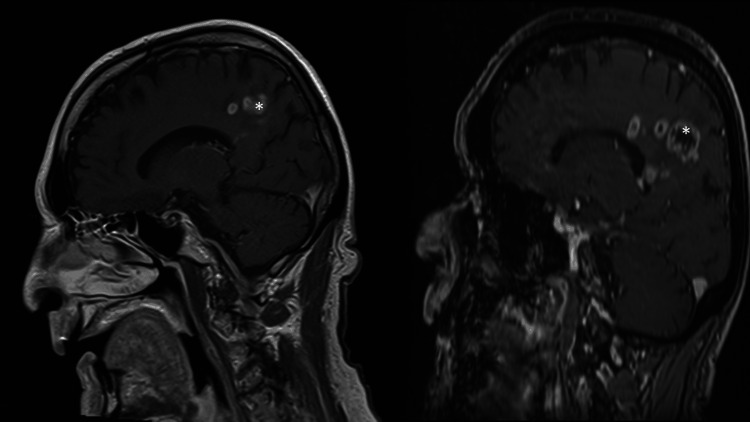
Sagittal MRI T1 with contrast scans of the initial presentation of the patient with right frontoparietal intraparenchymal lesions (LEFT), which showed significant growth over a one month time frame (RIGHT) Tumor labeled with (*)
